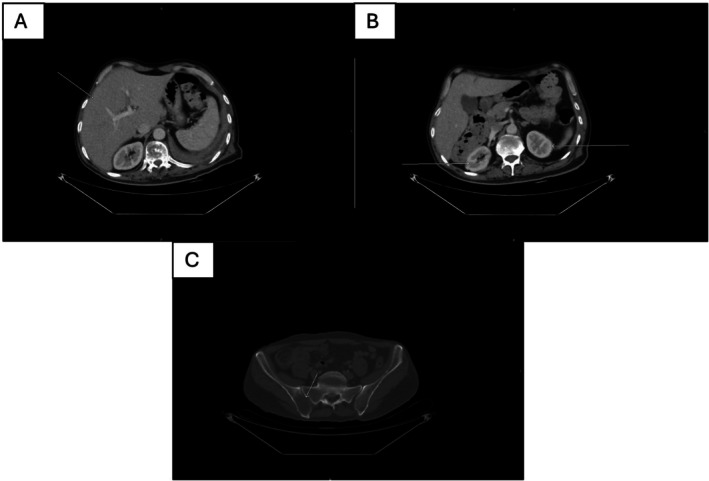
Contrast‐enhanced axial CT images demonstrating the absence of focal solid‐organ metastases and the presence of osseous involvement: (A) homogeneous hepatic parenchyma without focal lesions, (B) normal enhancement of both kidneys with no focal renal masses and (C) a lytic lesion involving the pelvic bone (arrow), consistent with metastatic disease.
